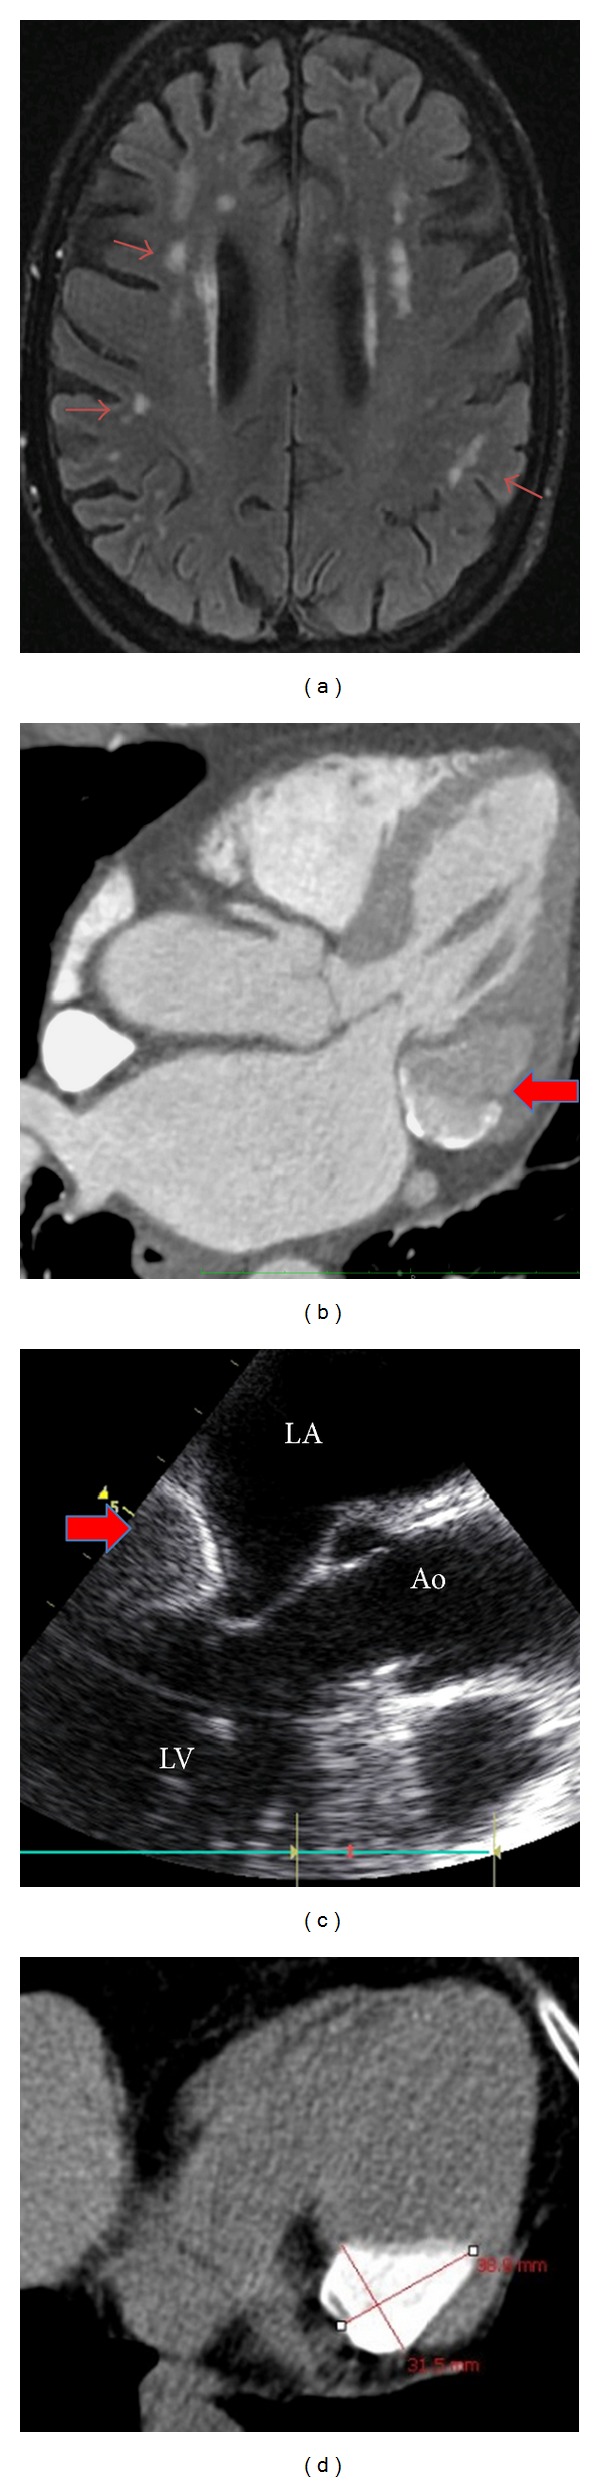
(a) Cerebral MRI shows multiple infarcts. ((b) and (d)) Cardiac CT shows a large calcified tumor at the insertion of the mitral annulus. (c) Transesophageal echocardiography shows a hyperechogenic, unobtrusive tumor fixed to the posterolateral wall of the left ventricle.
